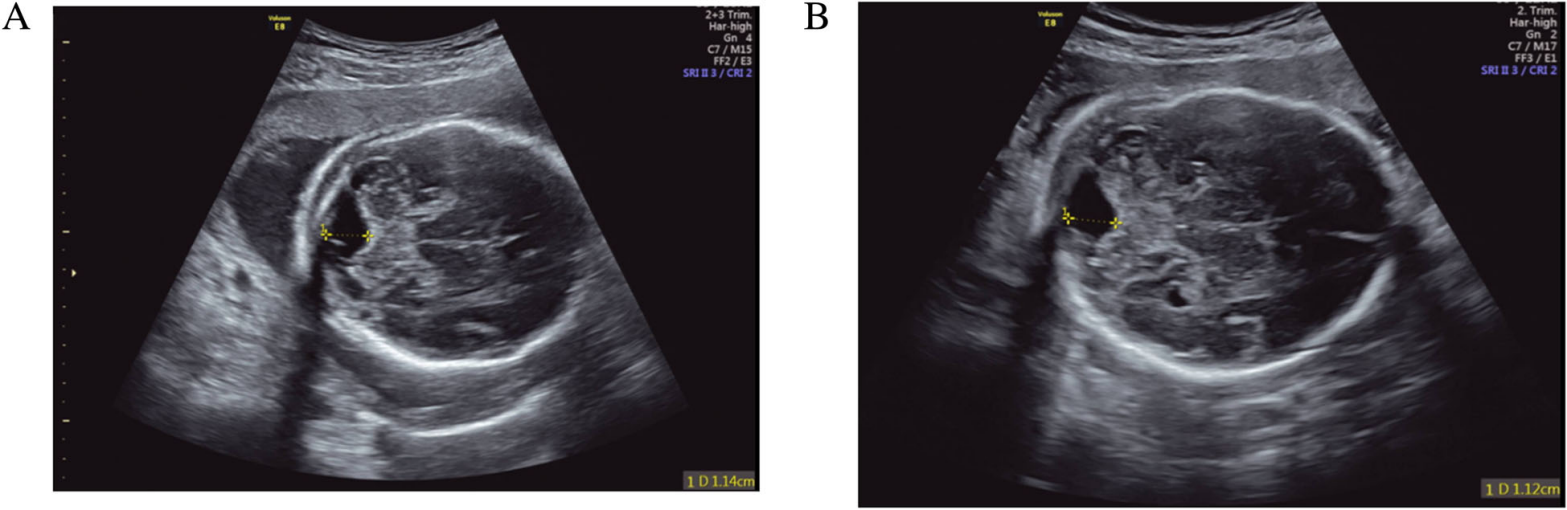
Ultrasound examination images. a Ultrasound examination result at 22 weeks. The width of the posterior cranial fossa was 11.4 mm. b Ultrasound examination results at 28 weeks. The width of the posterior cranial fossa was 11.2 mm
